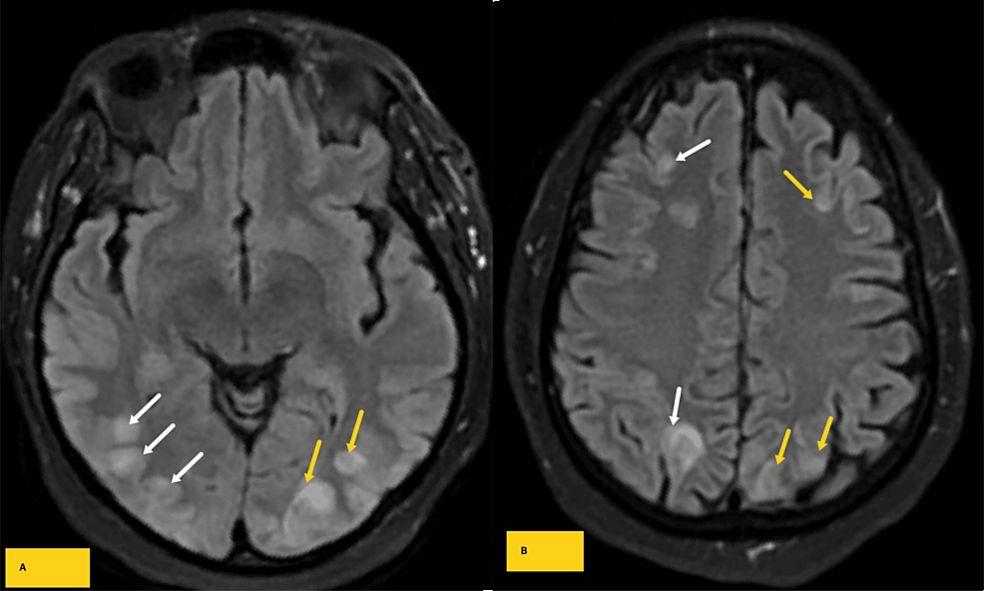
Axial fluid inversion recovery sequence (FLAIR) MR images through parieto-occipital lobes (A) and high frontoparietal (B) lobes extending to the posterior frontal lobes demonstrating multifocal subcortical areas of inversion recovery sequence (FLAIR) signal intensities in the parieto-occipital and high frontal subcortical areas corresponding to findings on the same day CT and compatible with posterior reversible encephalopathy syndrome.
